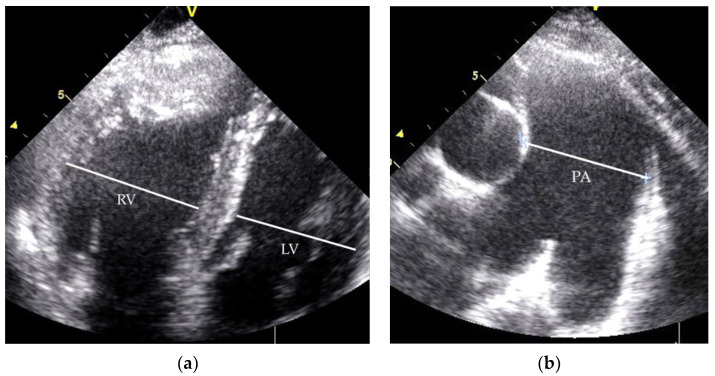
Patient 2 echocardiogram: (a) dilated right ventricle; (b) dilated pulmonary artery. RV—right ventricle; LV—left ventricle; PA—pulmonary artery.
